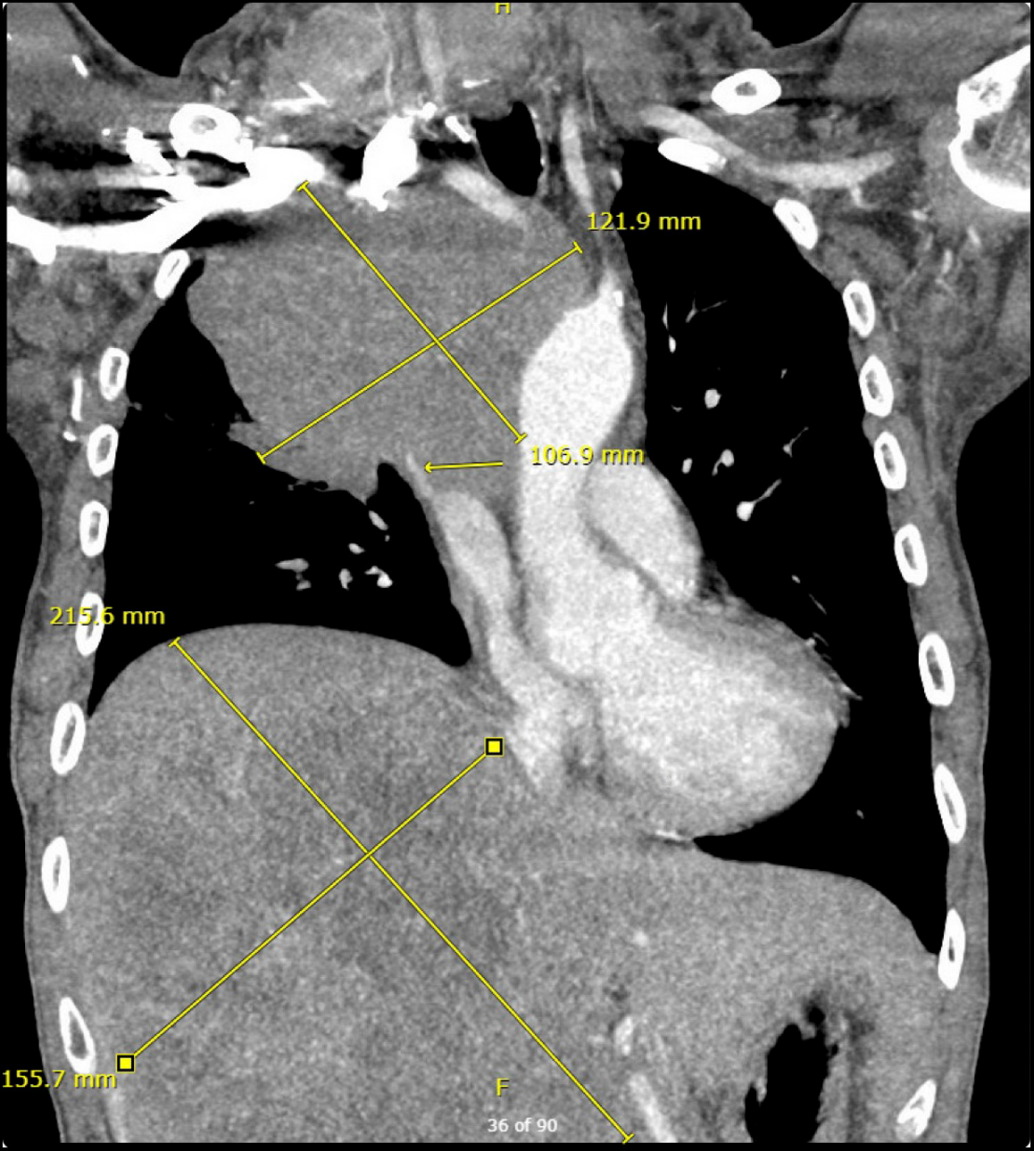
CT Chest/Abdomen/Pelvis with contrast showing a 12 × 10 cm mass occupying the right upper lobe compressing the SVC and a second mass in the liver measuring 21.5 × 15.5 cm compressing the IVC.
